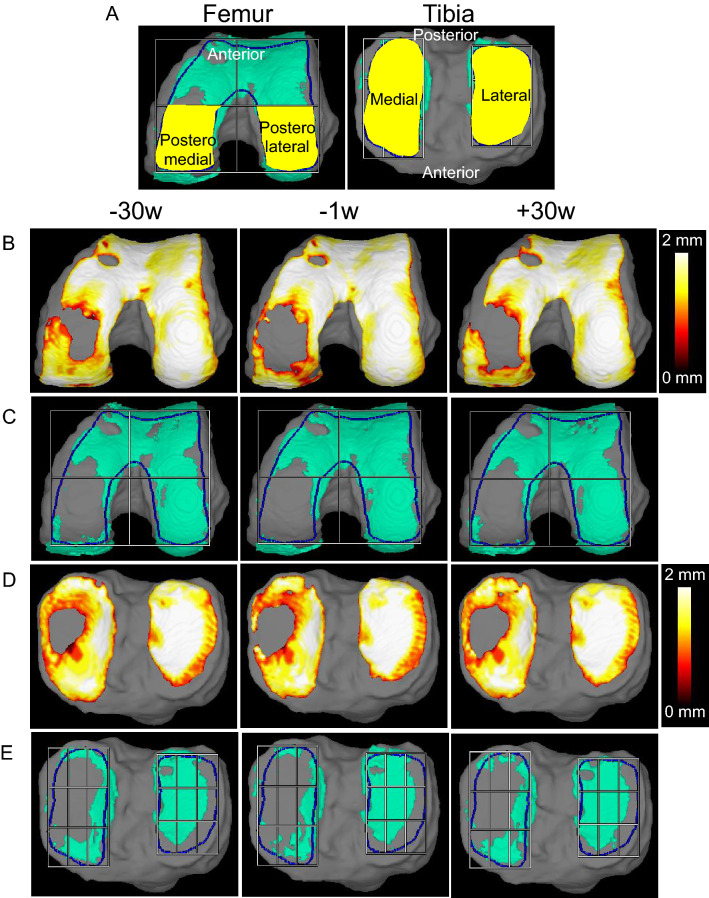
Reconstructed 3D MRI for cartilage in patient 01. (A) Orientation and region of interest (ROI) (yellow area). (B) Cartilage thickness mapping of the femur. (C) Projected cartilage area (thickness ≥ 1.5 mm) of the femur. (D) Cartilage thickness mapping of the tibia. (E) Projected cartilage area (thickness ≥ 1.5 mm) of the tibia.
